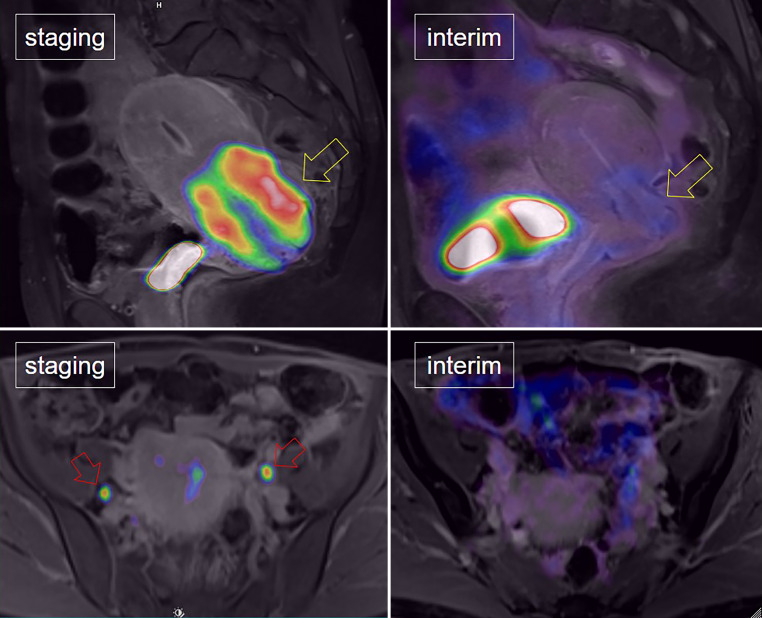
In a patient with cervical cancer, a complete metabolic response was observed. This was characterized by the complete disappearance of FDG uptake in both the primary cervical cancer lesion (yellow arrow) and the bilateral pelvic lymph node metastases (red arrows)
